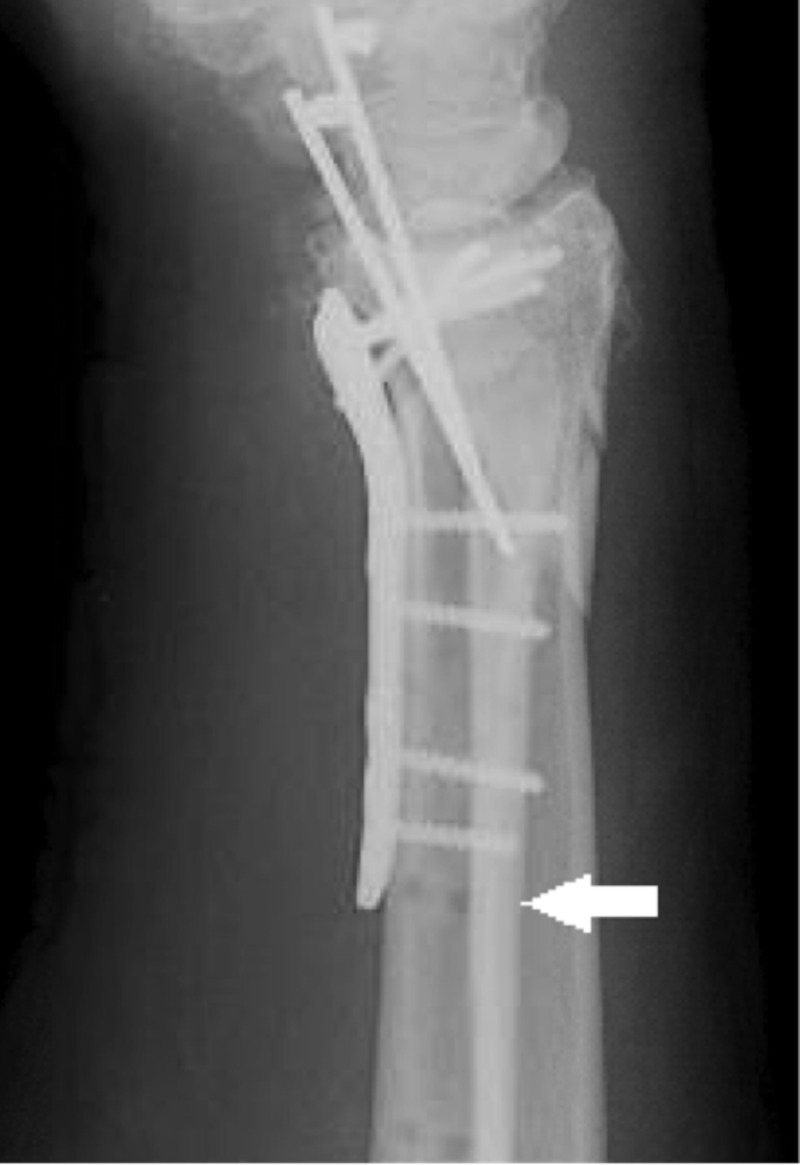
Lateral wrist X-ray demonstrating overlap of the volar plate with the track from the former pin site (white arrow).
